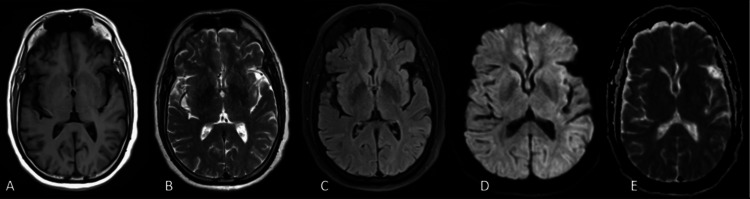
(A) T1-weighted imaging; (B) T2-weighted imaging; (C) T2-FLAIR imaging; (D) DWI; (E) Corresponding ADC map shows no significant abnormality FLAIR: Fluid-attenuated inversion recovery; DWI: Diffusion-weighted imaging; ADC: Apparent diffusion coefficient
